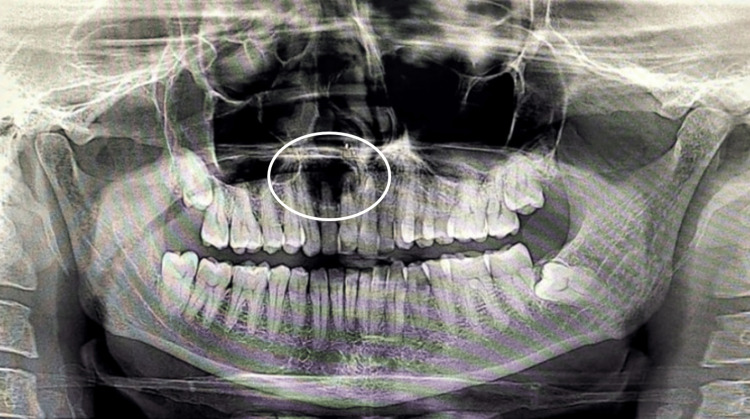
Preoperative OPG Showing periapical radiolucency in relation to 11,12 OPG: Orthopantogram.
